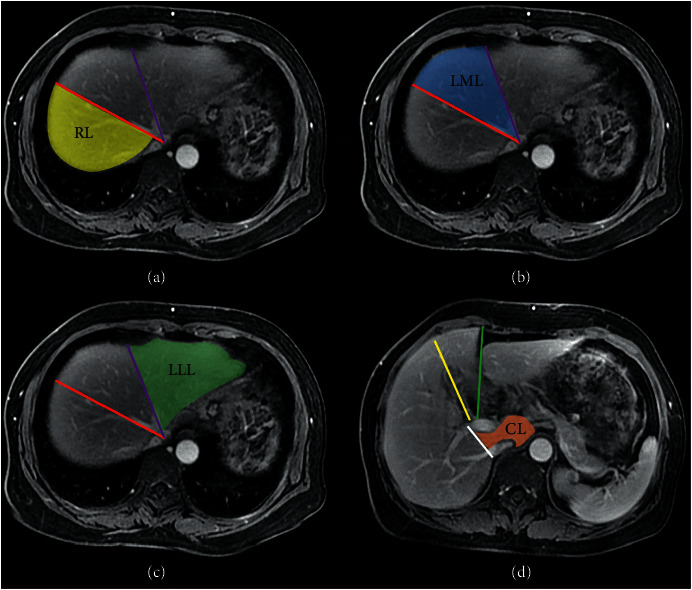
For the division of liver lobes on the axial-enhanced portal-venous phase magnetic resonance images, the middle liver vein (red line, (a)) is used as a landmark to differentiate the right liver lobe (RL) from the left liver lobe. The left liver vein (purple line) is used as a landmark to differentiate the left medial liver lobe (LML, (b)) from the left lateral liver lobe (LLL, (c)). The line linking the right branch of the portal vein to the inferior vena cava (white line) is used as a landmark to differentiate caudate lobe (CL) from RL (d).
